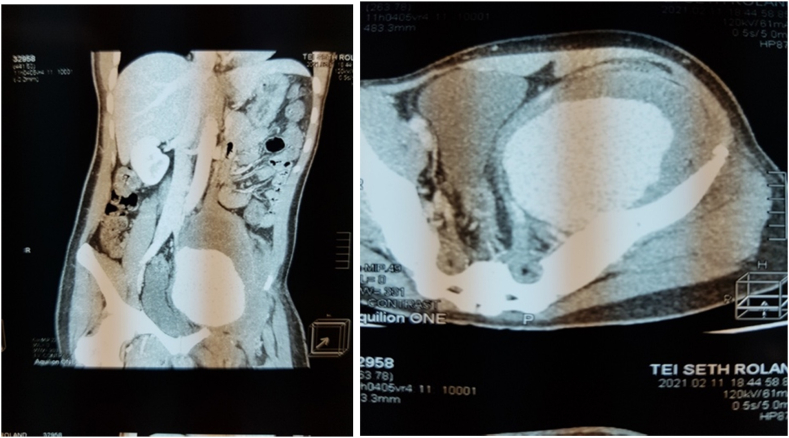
Pre-operative Computerized tomography angiogram (CTA) showing a huge left internal iliac artery aneurysm.
